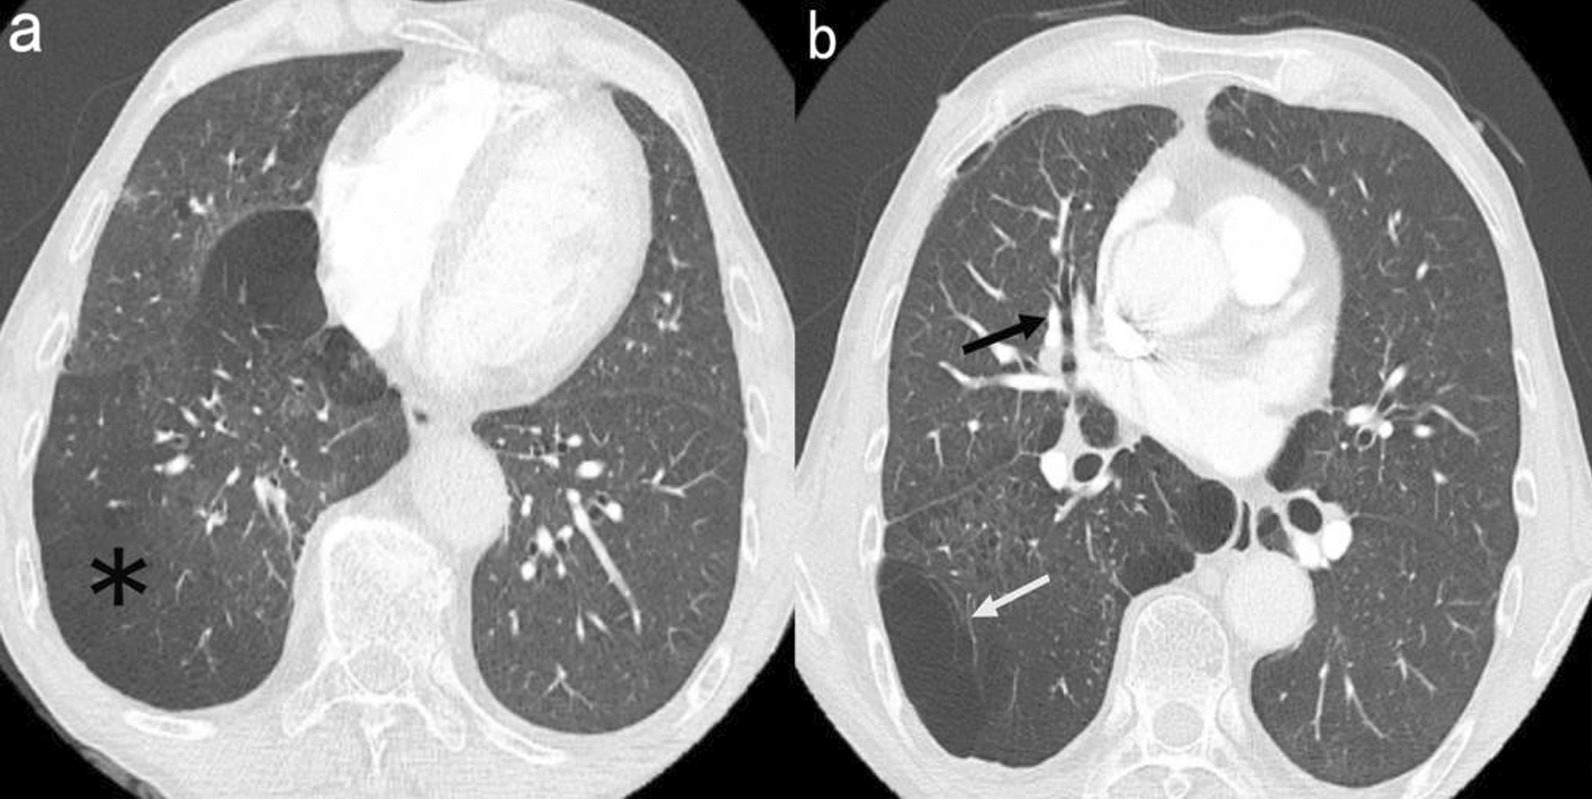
Axial images from chest computed tomography; a chest computed tomography scan presented patchy area of air trapping (_*_), b chest computed tomography scan revealed diffuse bronchial wall thickening with bronchiectasis (black arrow), local emphysema (white arrow), and leaf interval thickening; these findings are consistent with bronchiolitis obliterans, and the other typical sign of bronchiolitis obliterance, mosaic perfusion pattern, was not observed
